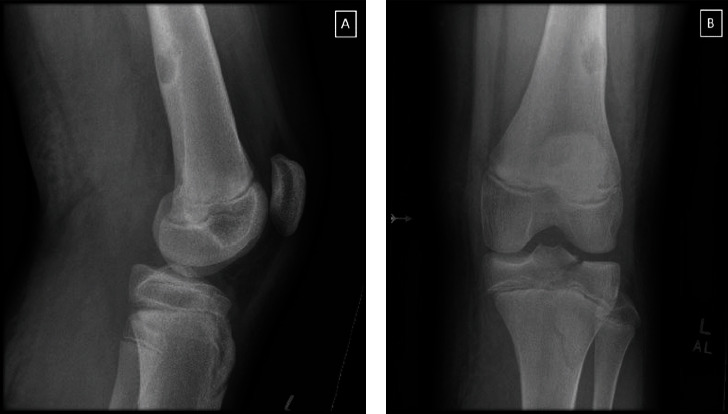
Knee radiograph demonstrating a displaced Salter-Harris III fracture of the proximal tibial epiphysis with incidental benign cortical lesion in the distal femur. (a) Lateral; (b) anterior posterior.
